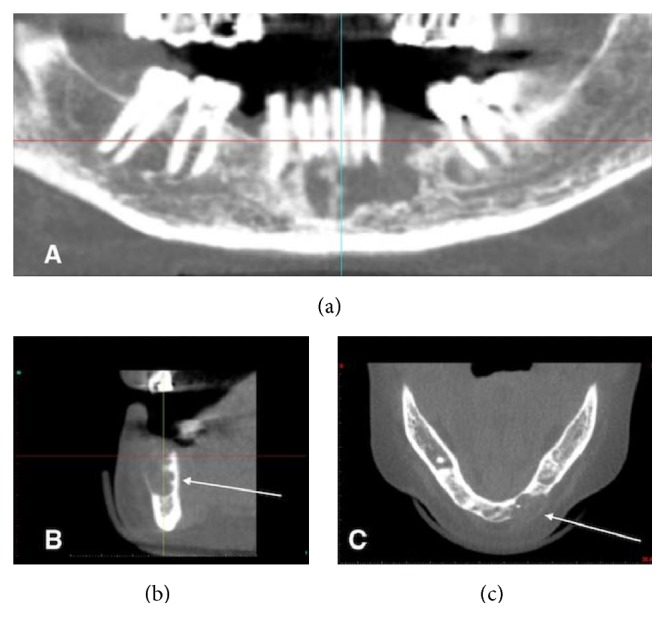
Relapse of the lesion. (a) The next examination, three months later, revealed the presence of a radiolucent cyst-like lesion in the mandible with mildly defined and irregular borders. (b) The CT radial view showed lingual cortical plate with a polylobate appearance and erosion of the buccal cortical plate (arrow). (c) The CT axial view highlighted the buccal erosion of the cortical plate (arrow).
